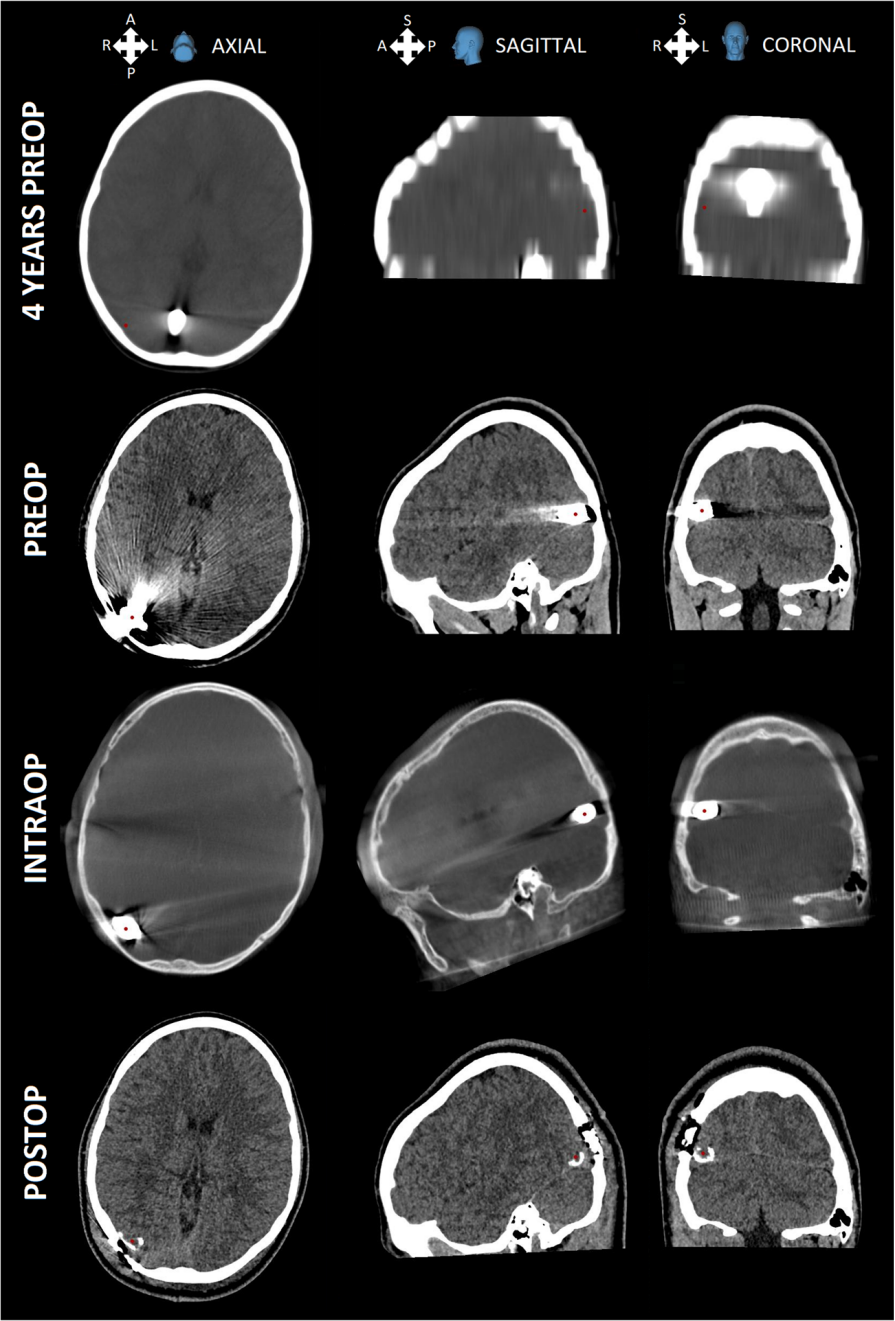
Upmost are the first CT images taken 4 years before surgery. Below that are CT images taken 4 months before surgery showing the bullet movement from midline to more lateral position. Next, intraoperative O-arm images present the situation at the time of surgery, and, finally, postoperative CT images reveal the calcified residual of the bullet’s capsule. Images are fused together with Medtronic StealthStation S8 and a red dot is placed in the centre of the bullet
